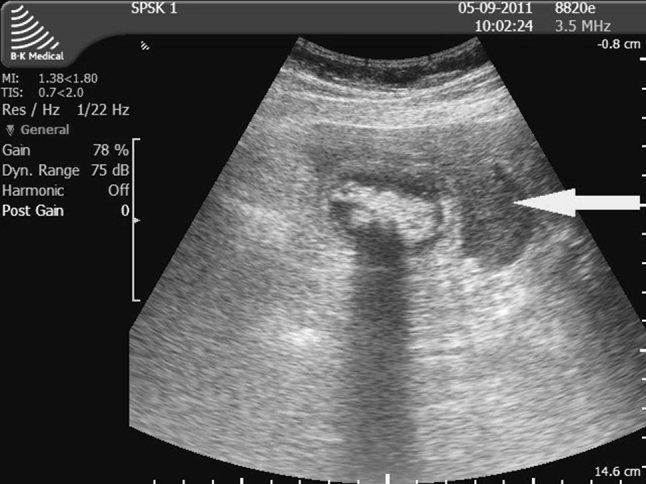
Ultrasound findings in acute cholecystitis that could affect the outcome of surgery: a severe inflammation and thickening of the gallbladder wall; on the liver side a fluid collection (pericholecystic abscess) is visible
